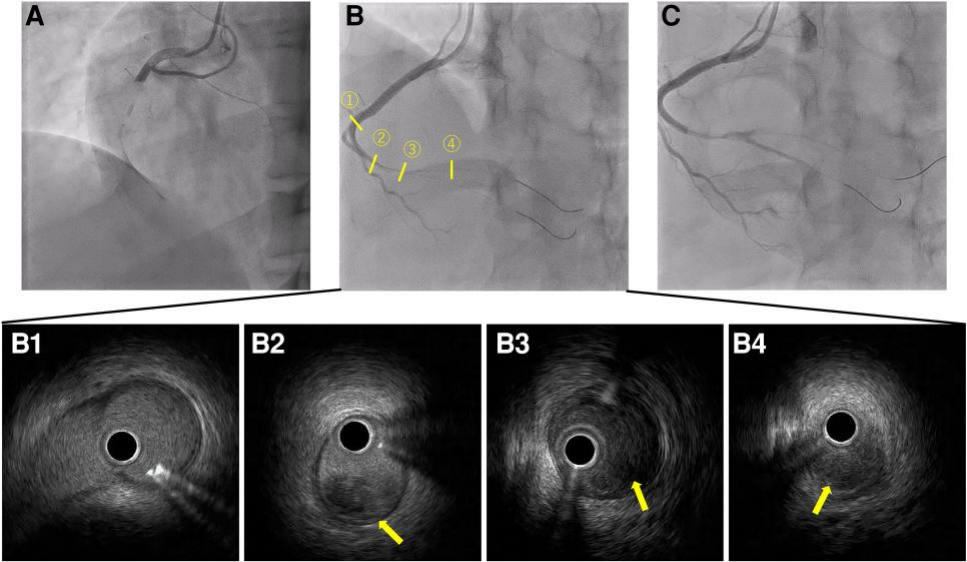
Second coronary angiography, percutaneous coronary intervention, and intravascular ultrasound (*A*) coronary angiography showed occlusion from the proximal portion of right coronary arteries, and no contrast of the distal right coronary arteries could be observed. (*B*) Coronary angiography and intravascular ultrasound findings after thrombus aspiration. The arrows on the intravascular ultrasound images indicate areas of low brightness within blood vessels, representing red thrombus. Large amounts of red thrombus filled the mid to distal portion of right coronary arteries. (*C*) Coronary angiography after percutaneous coronary intervention. Balloon dilation and thrombus retrieval were performed, but the thrombus was too massive for complete revascularization.
